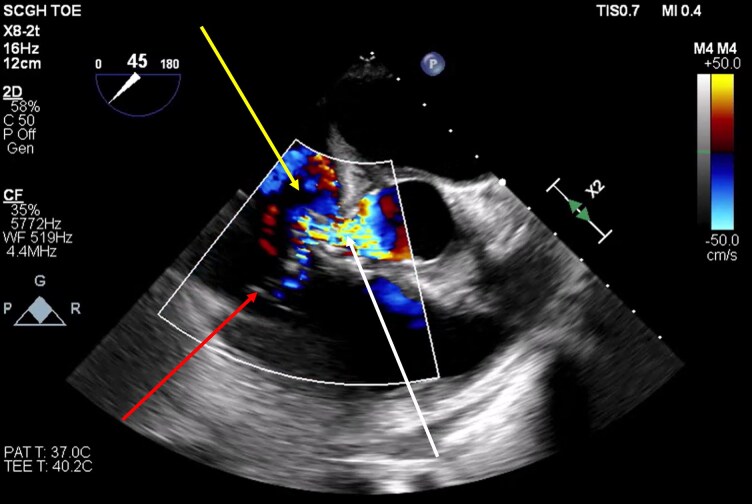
Intraoperative transoesophageal echocardiogram. Mid-oesophageal aortic valve short-axis view (45°): shows the jet’s origin near the non-coronary cusp (white arrow) and its direction into the right atrium (yellow arrow), confirming the presence of a Gerbode defect. The tricuspid valve is indicated by a red arrow.
